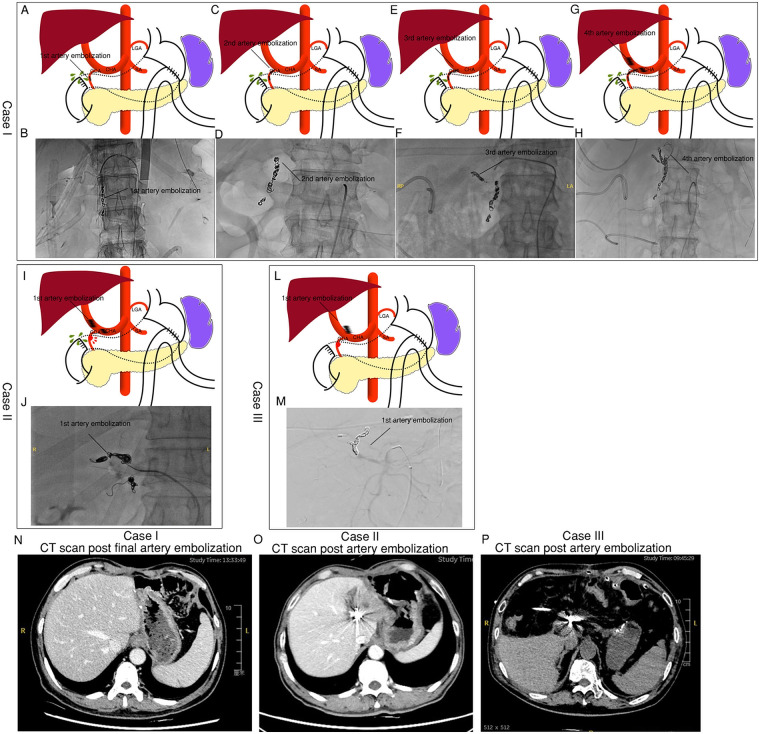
Demonstration of two gastric cancer cases with radical D2 lymphadenectomy and Billroth II, which were diagnosed as duodenal stump leakage and delayed massive artery hemorrhage, followed by emergent artery embolization of the common hepatic artery, proper hepatic artery, and gastroduodenal artery. (A) Demonstration of artery embolization and duodenal stump leakage site in Case I; (B) artery hemorrhage and embolization of first DSA in Case I; (C,D) second artery hemorrhage and embolization of DSA in Case I; (E,F) third artery hemorrhage and embolization of DSA in Case I; (G,H) fourth artery hemorrhage and embolization of DSA in Case I; (I,J) artery hemorrhage and embolization of DSA in Case II; (L,M) artery hemorrhage and embolization of DSA in Case III; (N–P) DSA postoperative CT scan post-final artery embolization in Cases I (N), II (O), and III (P).
